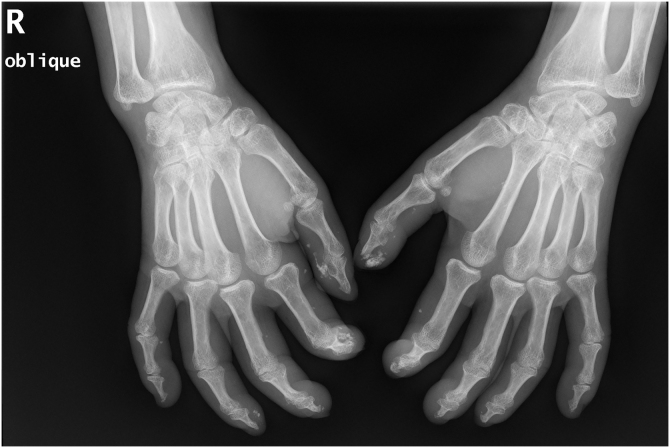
Hands simple radiography (oblique view) after 25 sessions of treatment with intralesional sodium thiosulfate.
